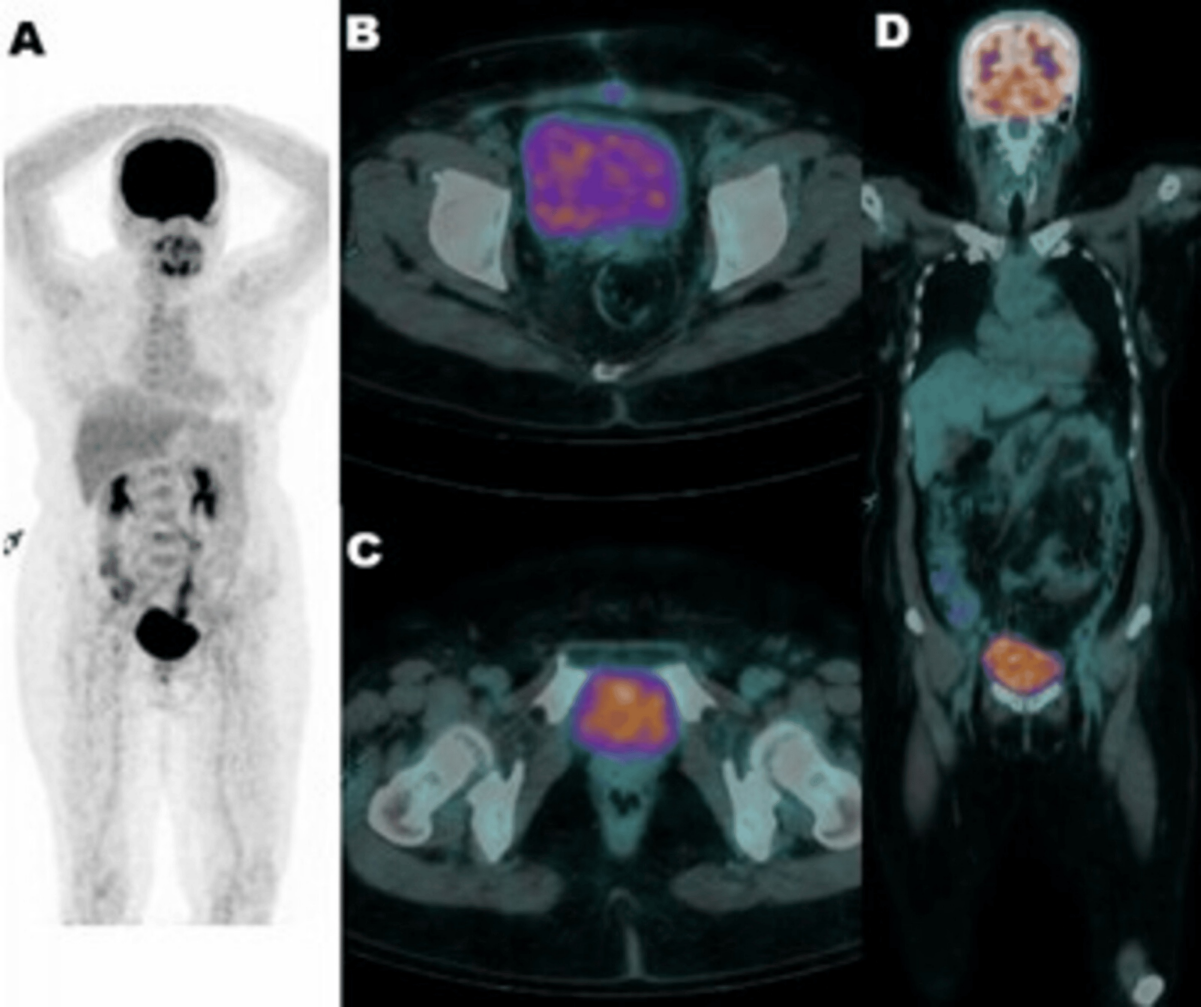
Baseline [18F] fluorodeoxyglucose positron emission tomography/computed tomography (PET/CT) performed for the detection of metastasis (A) maximum intensity projection, (B) pelvic transaxial PET/CT, (C) rectal transaxial PET/CT, and (D) head-abdomen coronal PET/CT.
